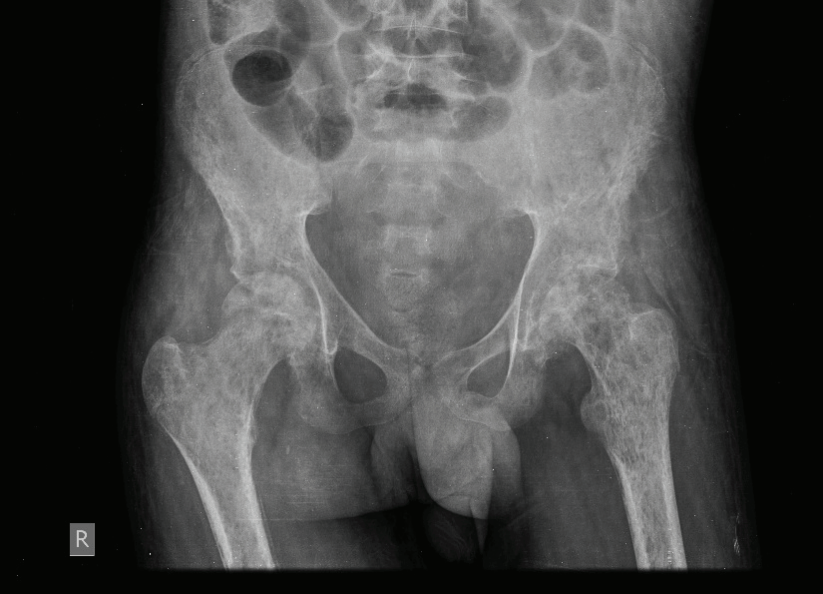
X-ray of the pelvis showed multiple permeative lesions involving the pelvic bones and the visualised proximal femurs.
